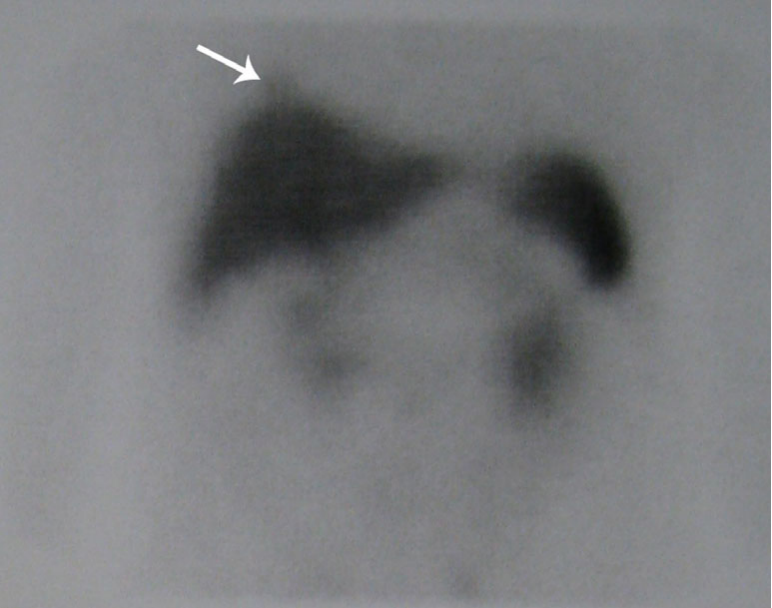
Technetium-99m-labeled octreotide acetate scintigraphy in spot abdominal view. This was performed 3 hours after injection of 15mCi technetium-99m-labeled octreotide acetate. There is a focal uptake in the lower lobe of our patient's right lung, just above his diaphragm, highly suggestive of an adrenocorticotropic hormone (ACTH)-producing bronchial tumor.
